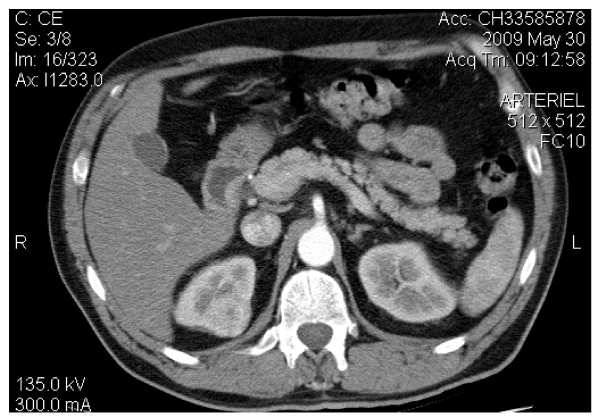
CT of the abdomen. Heterogeneous enhancing lesion in the upper pole of the right kidney without invasion to surrounding tissues.
